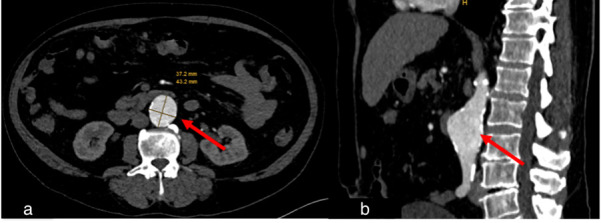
Case 5, 67-year-old male. A chest CT scan was performed to evaluate for pulmonary embolism after recovering from COVID-19. (a) Axial view, (b) sagittal view. As incidental finding, an abdominal aortic aneurysm was discovered (red arrows).
